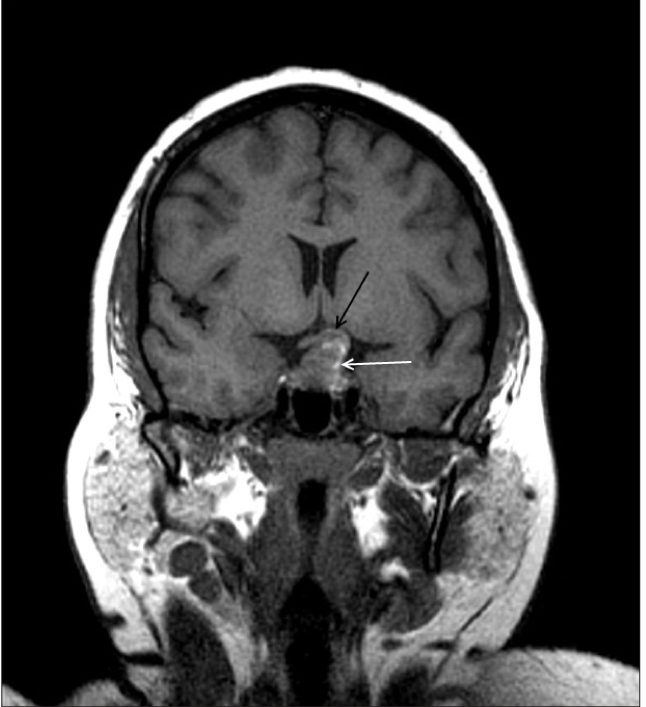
Coronal T1W MR image shows a predominantly isointense mass with few hyperintense areas (white arrow). It is bulging to left of midline and causing compression of left half of optic chiasma (black arrow)
